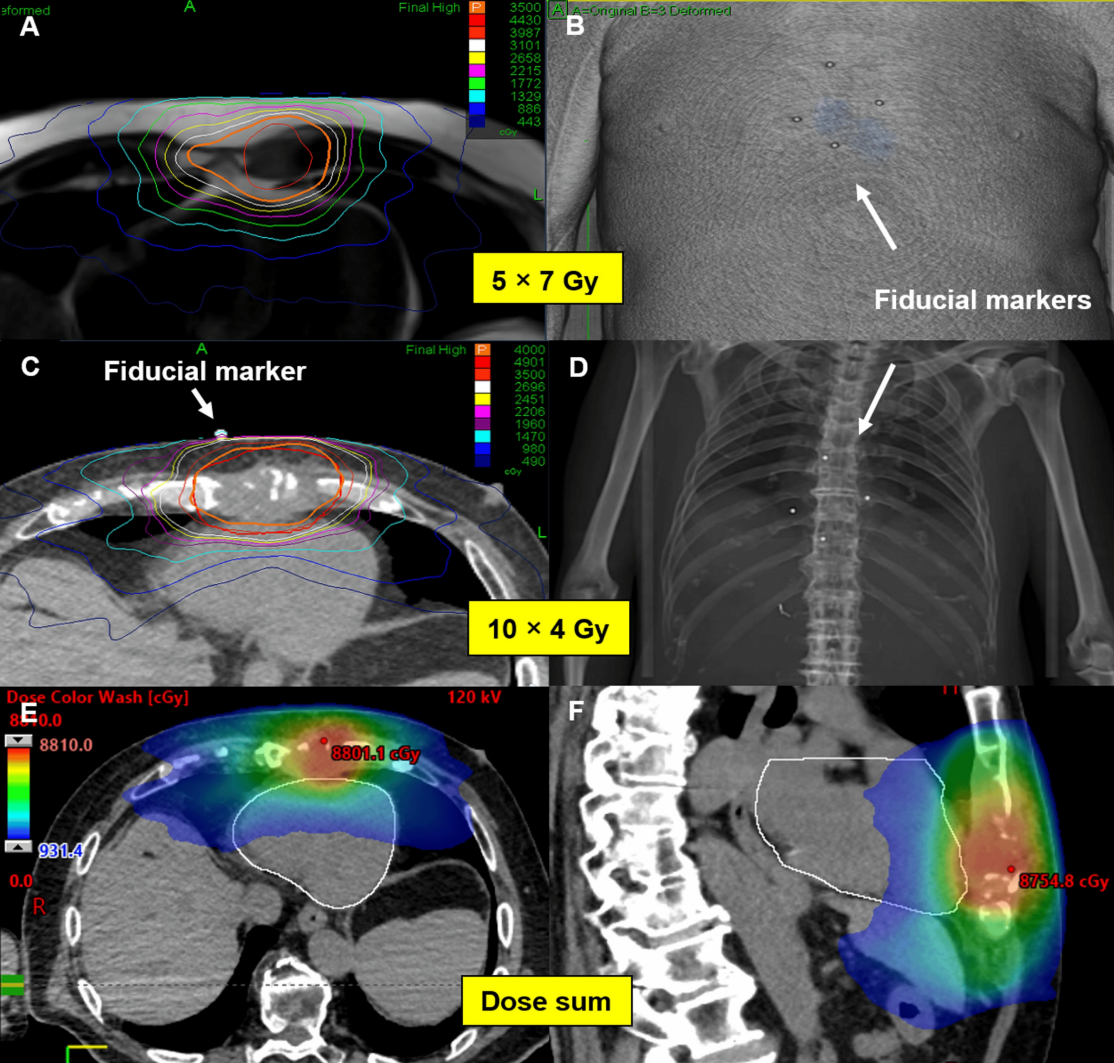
First SBRT with 5 × 7 Gy (A) and FMs placed in close proximity to the sternal lesion on the skin (B). Positioning of the FMs were marked with tattoos at planning CT to ensure consistent positioning of the FMs for each fraction. For the second SBRT (C) 10 × 4 Gy was delivered. Since the tattoos from the first treatment course were still visible, the FMs could be positioned at the exact same position for the second SBRT, as shown in the planning CT and DRR (C, D). The bottom two images (E, F) show a dose summation in color wash of both SBRTs. Notably, in this plan sum the mean and maximum heart doses were 11.3 Gy and 81 Gy, respectively, while 15 cc of the heart received 41.4 Gy, remaining within the D15cc dose constraint of 42 Gy in 15 fractions as recommended by Timmerman. SBRT = stereotactic body radiotherapy, FMs = fiducial markers, DRR = digitally reconstructed radiograph Timmerman [4]
